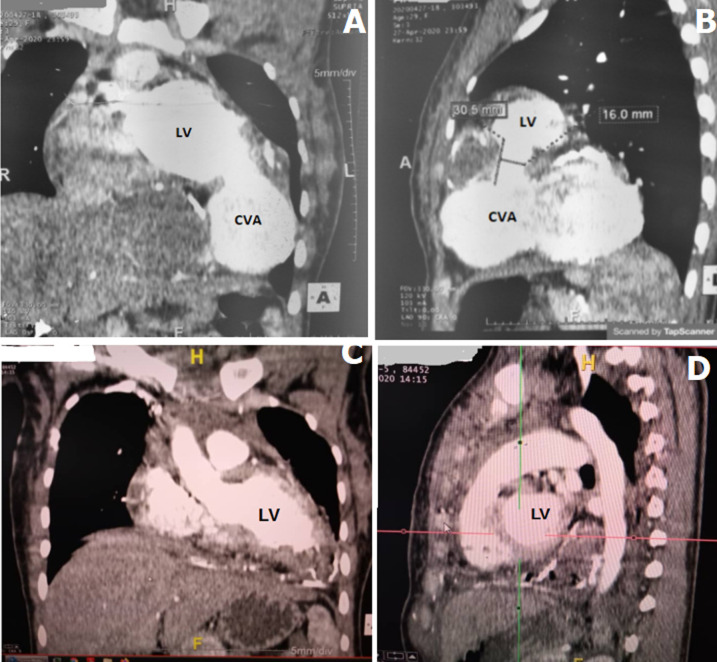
angio-computed tomography scan views: comparative aspects, preoperative (A, B), postoperative (C, D)
